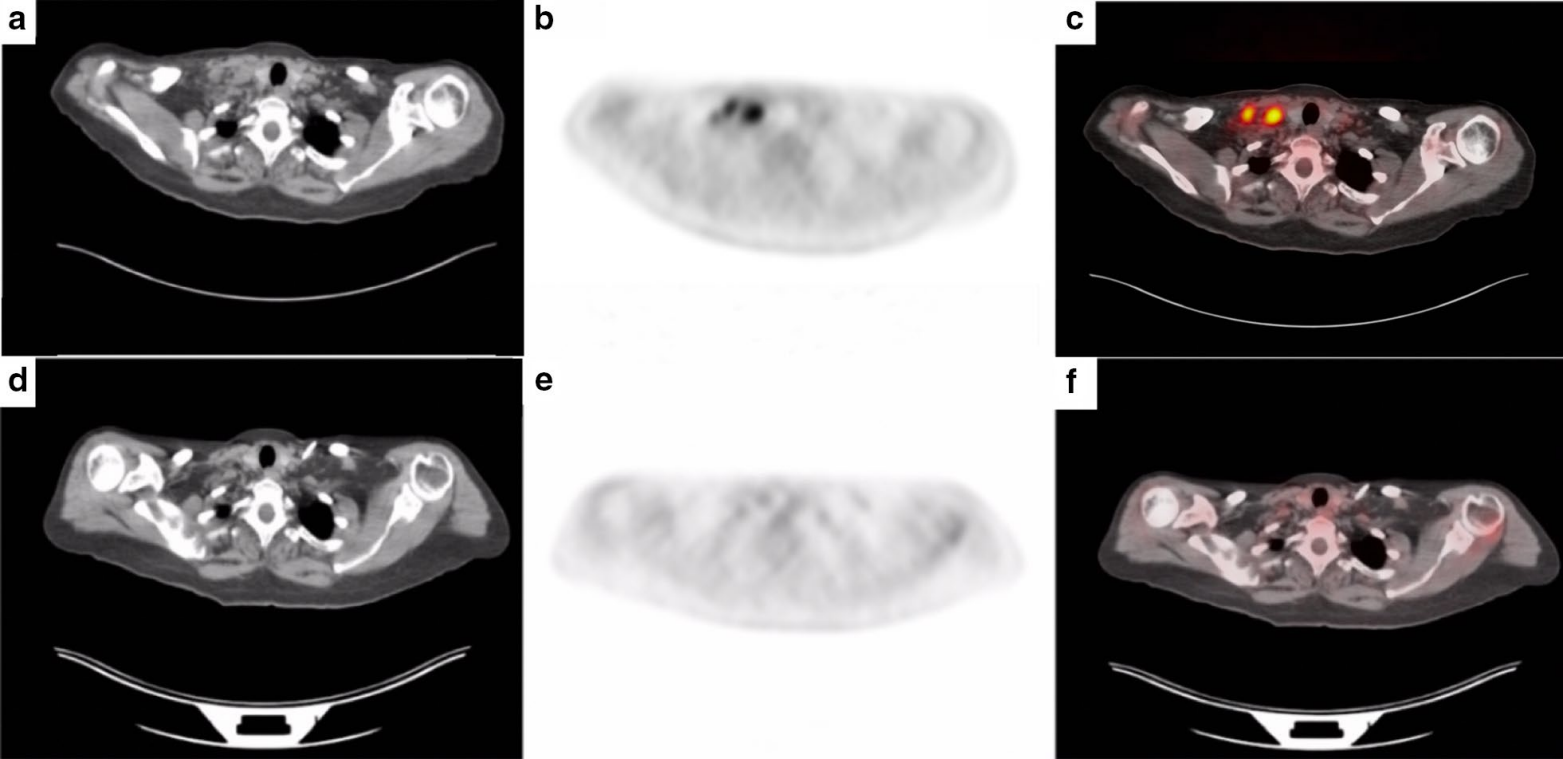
a–c consist of the CT, PET, and PET/CT scans, respectively, of a subject with multicentric Castleman Disease (MCD). High FDG uptake of lymph nodes can be visualized in the neck in b and c. d–f were taken 8 months after (a–c) and demonstrate decreased FDG-uptake in the same neck region, indicating that the subject’s Castleman disease treatment has been successful
